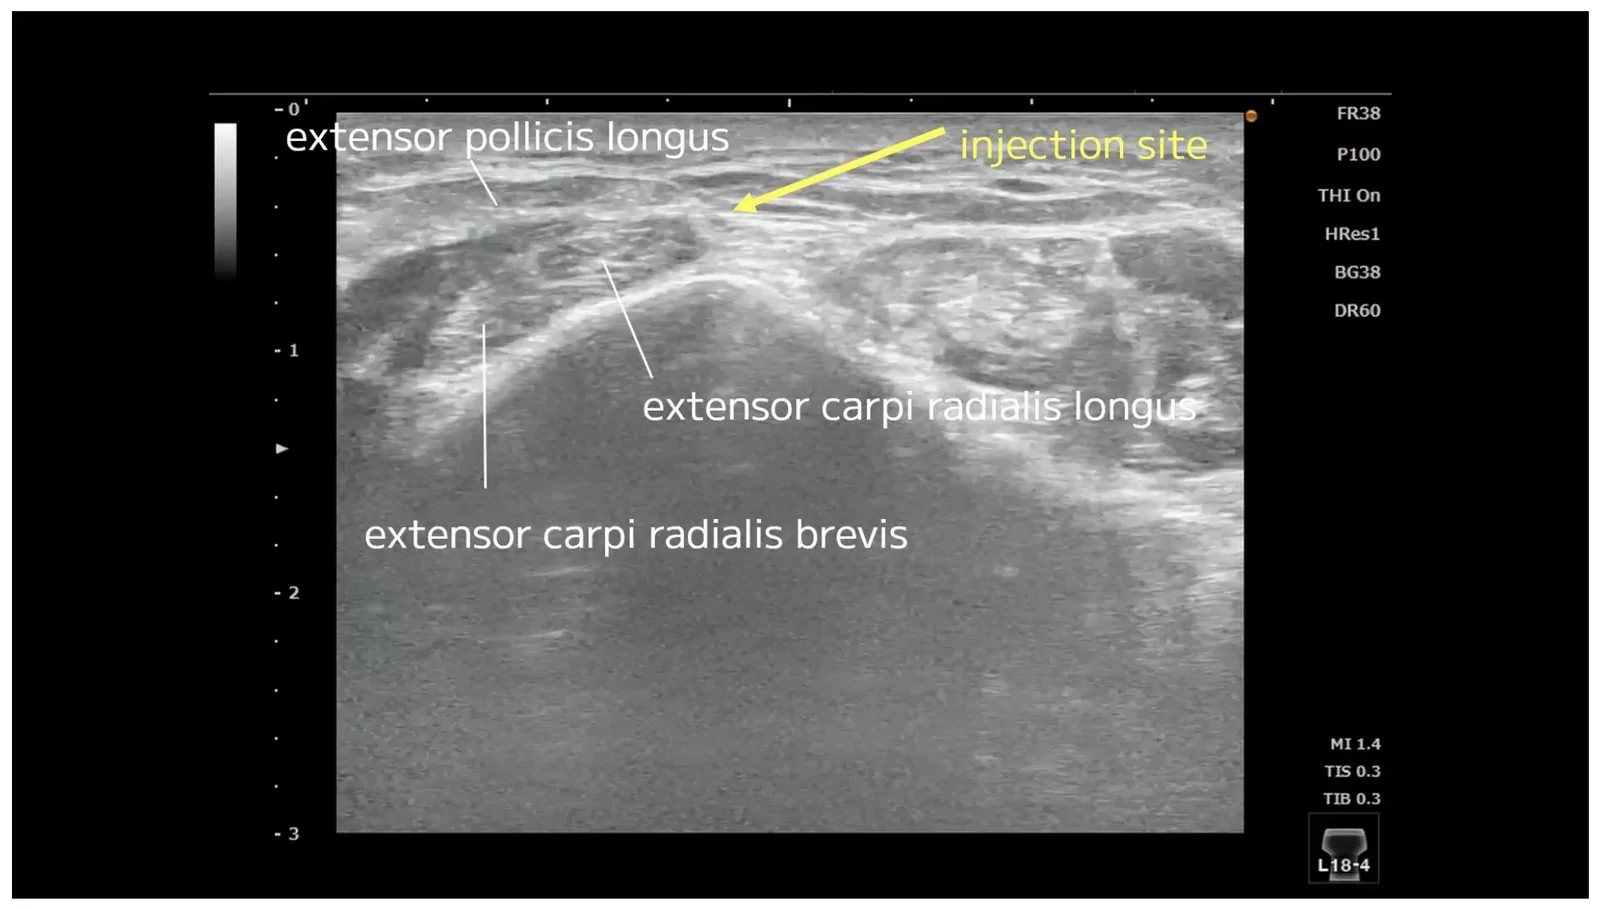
Point 4—second–third compartment intersection: ultrasound-guided fascia hydrorelease; yellow arrow indicates the injection site.
